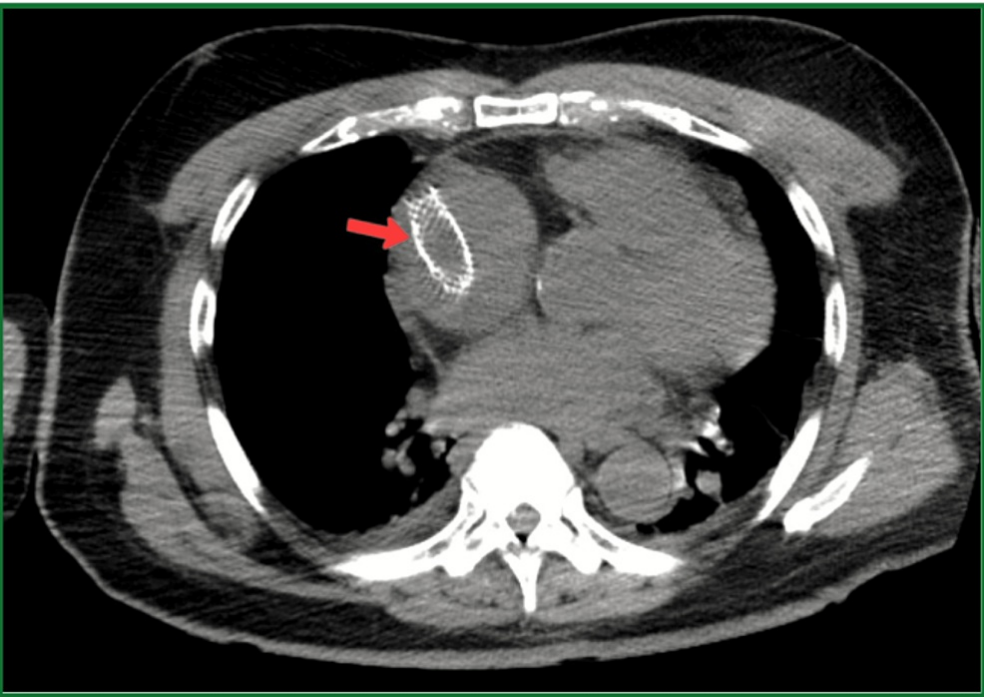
CT of the abdomen (transverse plane). Red arrow pointing at a stent in the right atrium
